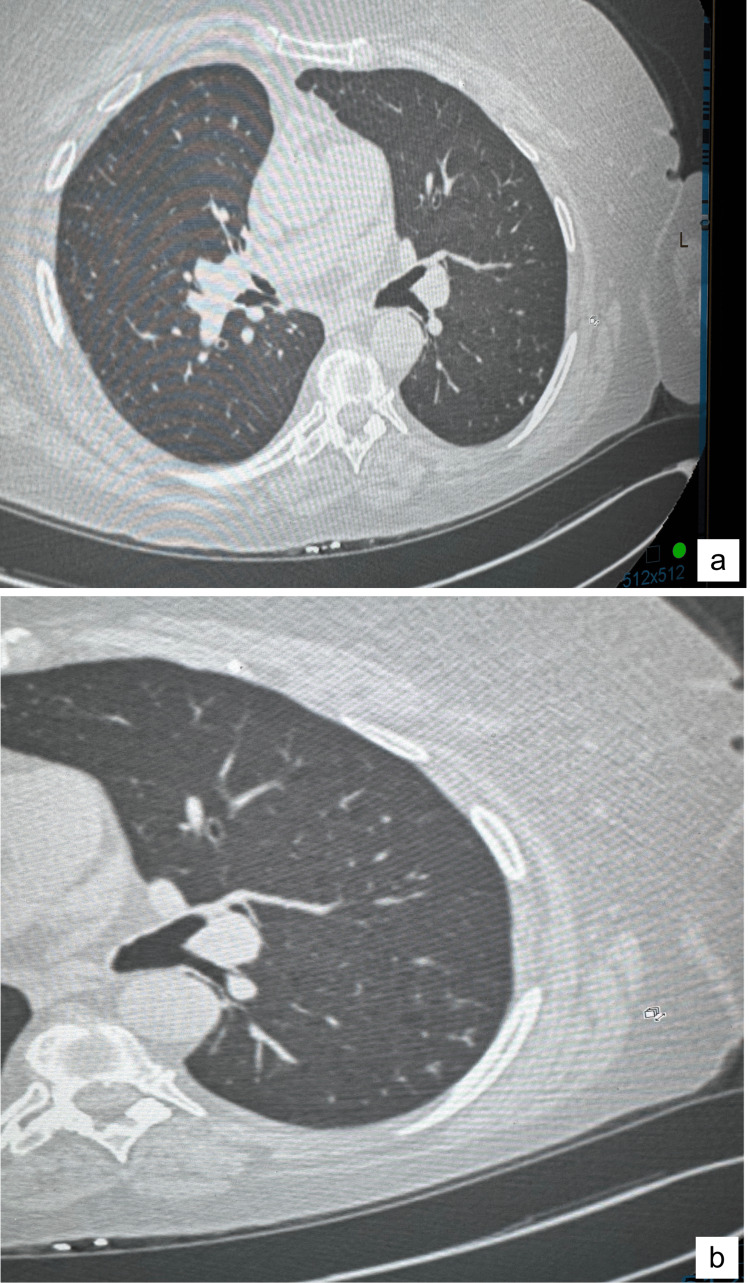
CT chest with coned-down view taken on 09/20/22 showing resolution of the cluster of micronodules and nodules in the left lung base after treatment CT: computed tomography.
